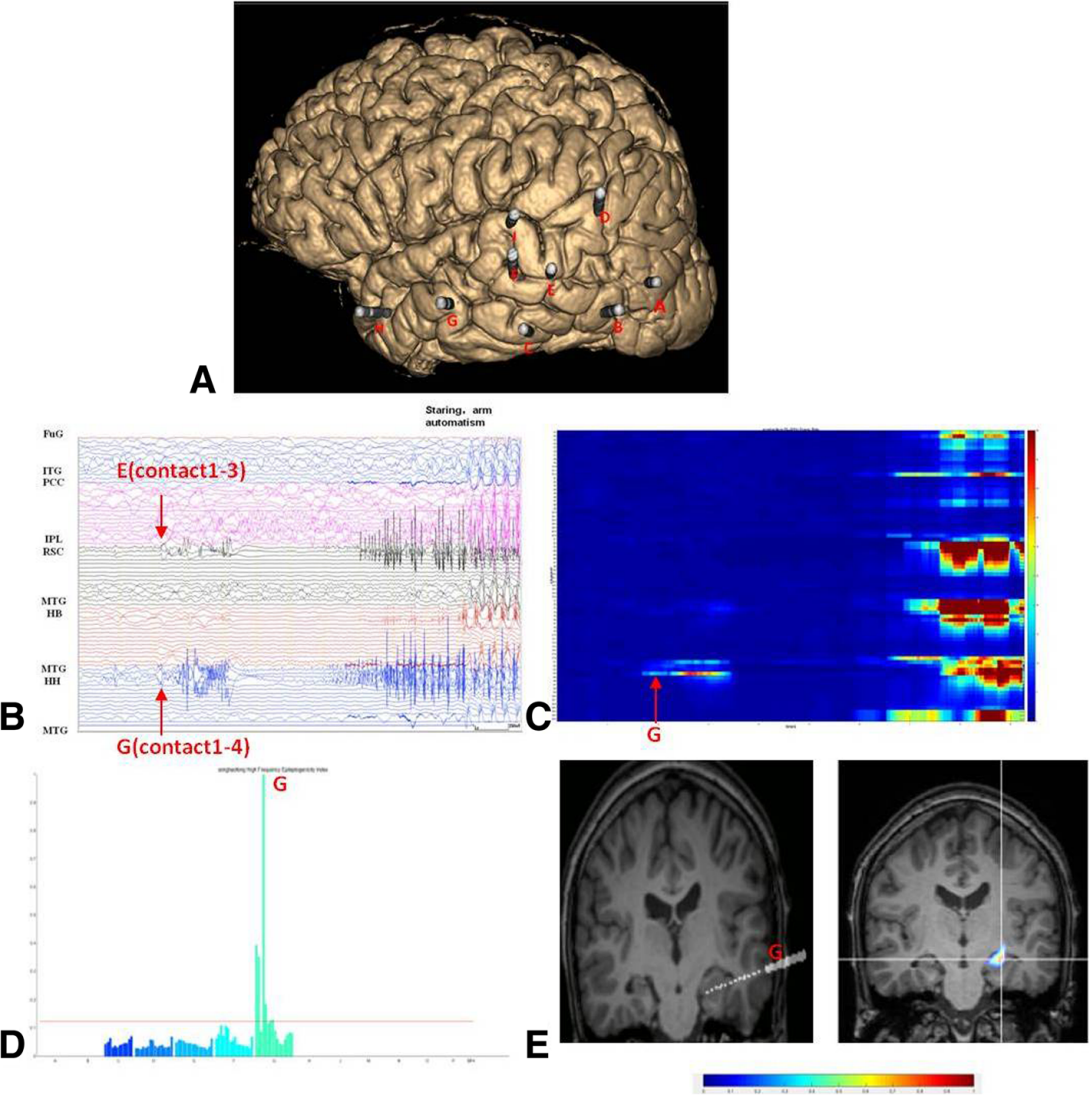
SEEG recordings of Patient 1. a Location of the SEEG electrodes shown on the 3D MRI image, The electrodes mainy explored the temporal occipital junction of left hemisphere. b From SEEG data, seizure started with a bursts of spikes in the head of hippocampus(internal leads of electrode G) and retrosplenial cortex(internal leads of electrode E);.c Quantification of the seizure onset confined to the electrode G. d The EI values quoted on the chart demonstrating maximal epileptogenicity in the electrode G. e EI analysis overlied MRI image, seizure onset was confined to the head of hippocampus. FUG:fusiform gyrus; PCC:posterior cingular cortex; IPL:inferior parietal lobule; RSC:retrosplenial cortex; MTG:middle temporal gyrus; HB:body of hippocampus; HH:head of hippocampus
